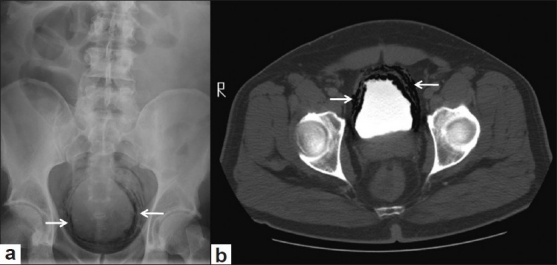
(a) Plain radiograph of the abdomen showing curvilinear lucencies in the region of urinary bladder in pelvis. (b) Axial CT section through pelvis confirms location of air within the bladder wall surrounding the contrast filled lumen
